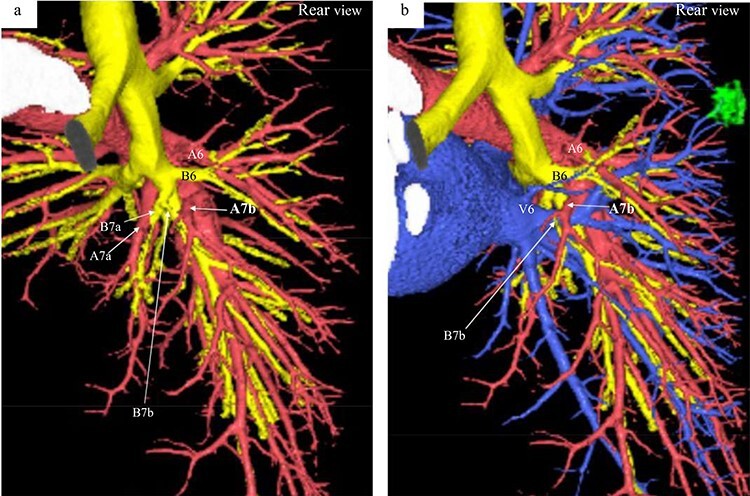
Three-dimensional CT angiography and bronchography images. (a) Rear-view CT angiography image of the PA and bronchi. (b) Rear-view CT image of the PA, PV, and bronchi. Reconstructed images of the pulmonary arteries (red), pulmonary veins (blue), bronchi (yellow) and tumor (green). A, pulmonary artery; B, bronchus; V, pulmonaryvein.
